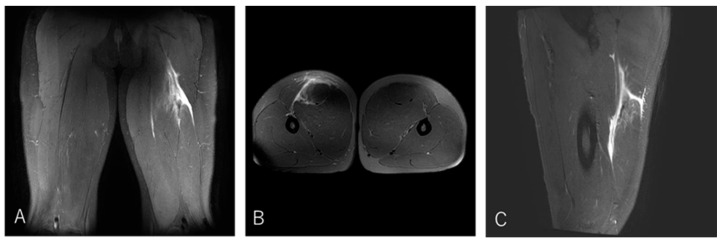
Case 2 MRI images: Conjoint tendon tear, Type I. (A) (Coronal image): Complete rupture of the conjoint tendon (CT). A haematoma is present where part of the CT is not visualised. The MTJ has retracted distally. (B )(Axial image): prone position, A defect on CT and haemorrhage are observed at the rapture site. (C) (Sagittal image): The ruptured distal CT tendon has retracted.
